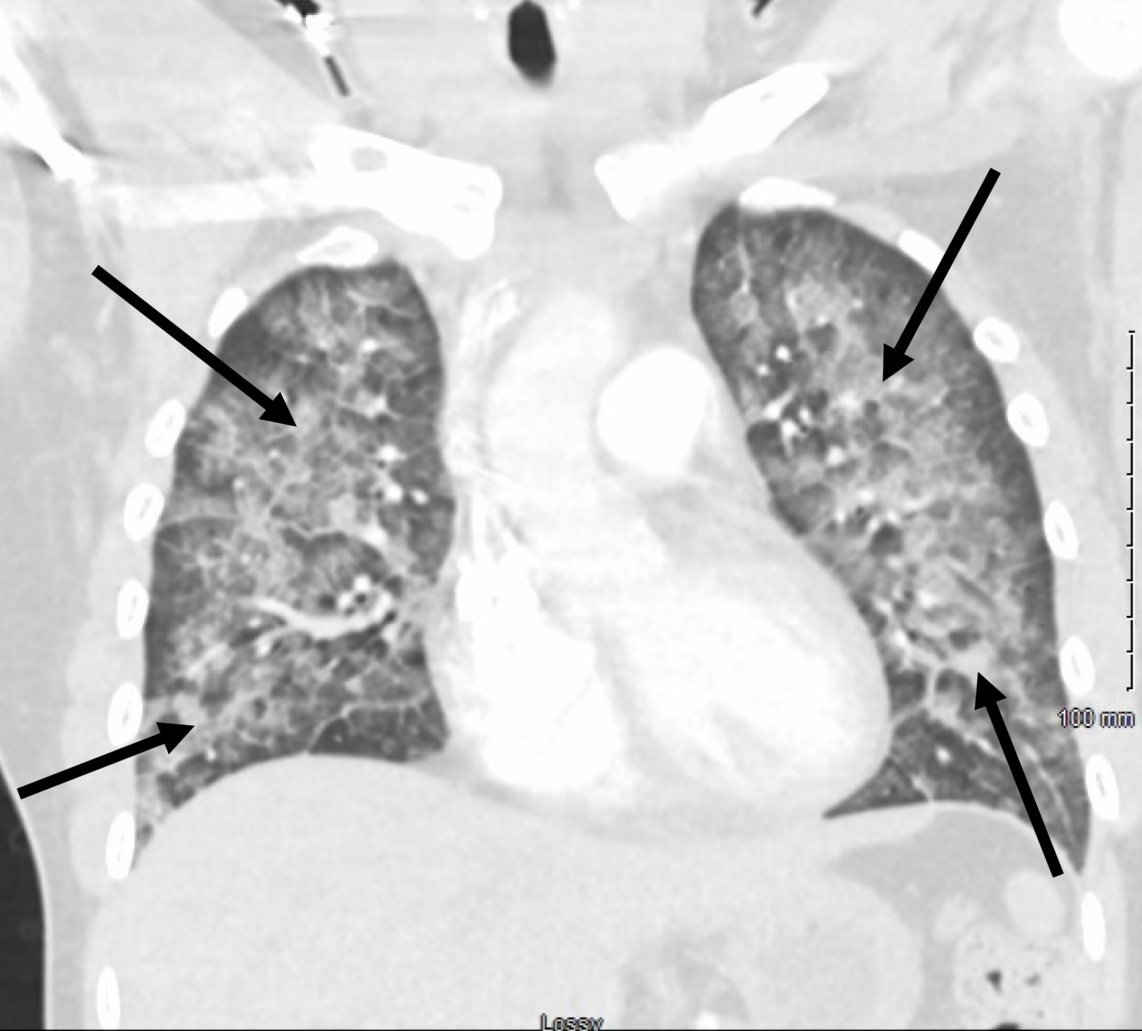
Chest CT (coronal plane image) showing diffuse bilateral pulmonary infiltrates (indicated by arrows).
